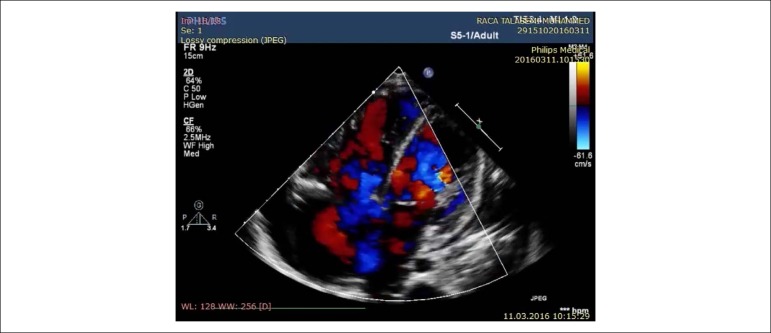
Transthoracic echocardiography from the apical four-chamber by color
